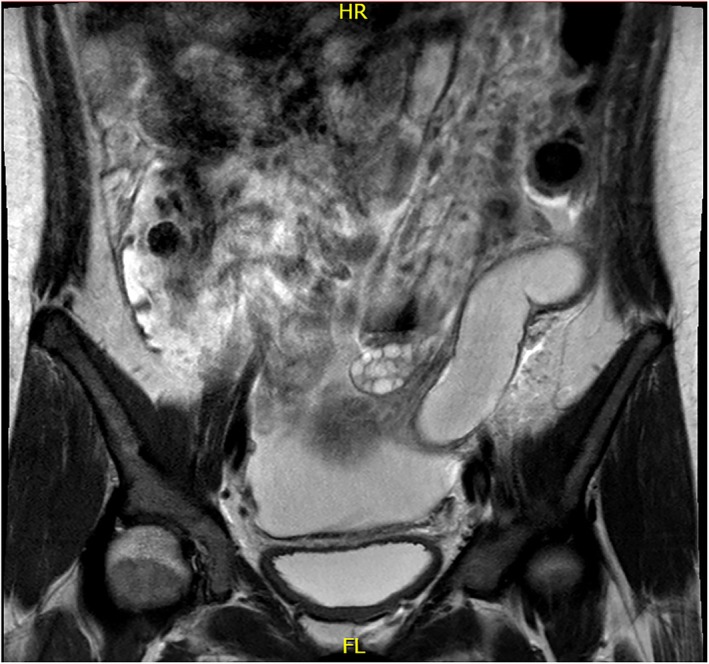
Multiparametric pelvic MRI, coronal T2W TSE HR sequence showing a partly tortuous tubular‐shaped structure in the left hemiabdomen compatible with ectatic left megaureter.
